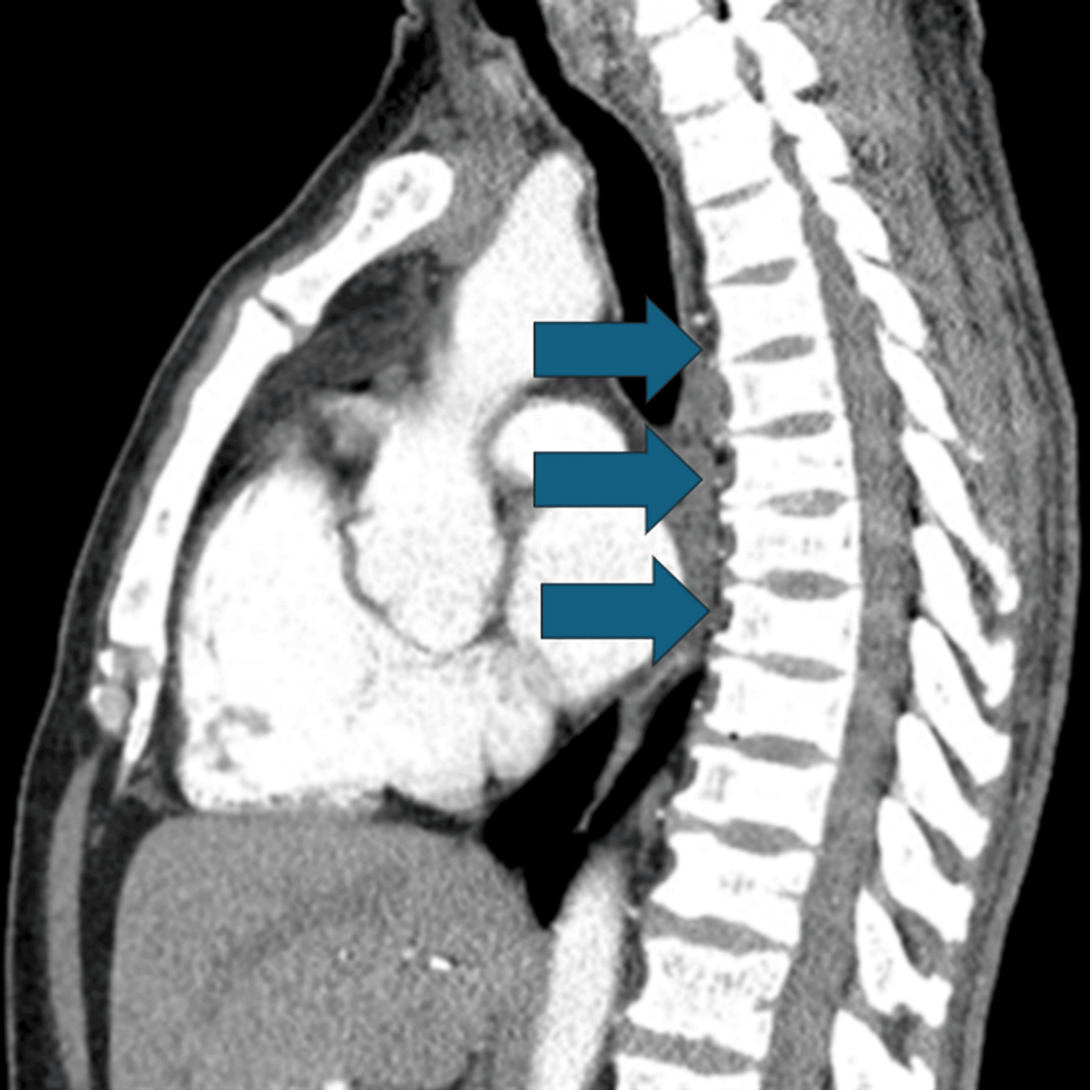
H-shaped vertebrae (Lincoln log vertebrae) were found on presentation with chest CT angiogram and are a finding seen in sickle-cell anemia; blue arrows highlight the affected vertebrae
